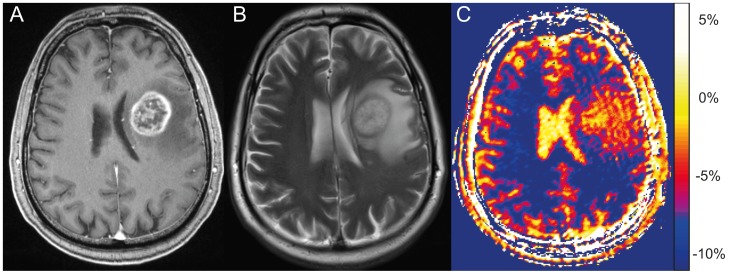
Peritumoral hyperintensity on NOE mediated CEST compared to standard MRI. Left frontal glioblastoma in a 59 year old man at 3 Tesla, CE-T1 (A) and T2-weighted images (B). On the selected slice the CEST contrast at 7 Tesla, based on MTR_asym_ (C), displays peritumoral hyperintensities at equal extent compared to the edema on T2-weighted images. In contrast to T2-weighted images, the CEST peritumoral hyperintensity displays an irregular border and subareas of different signal intensity.
